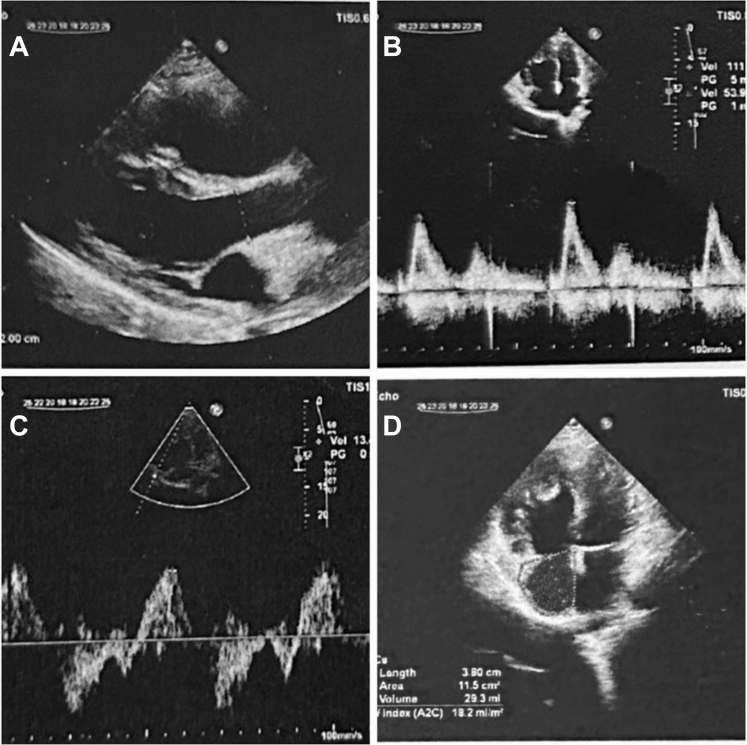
Preoperative Echocardiographic Assessment (A) Parasternal short-axis view of the right ventricular outflow tract showing abnormal pulmonary valve. (B) Continuous-wave Doppler across the pulmonary valve showing severe pulmonary regurgitation. (C) Tissue Doppler imaging at the tricuspid annulus assessing right ventricular systolic function. (D) Apical 4-chamber view showing right ventricular and right atrial dilation.
